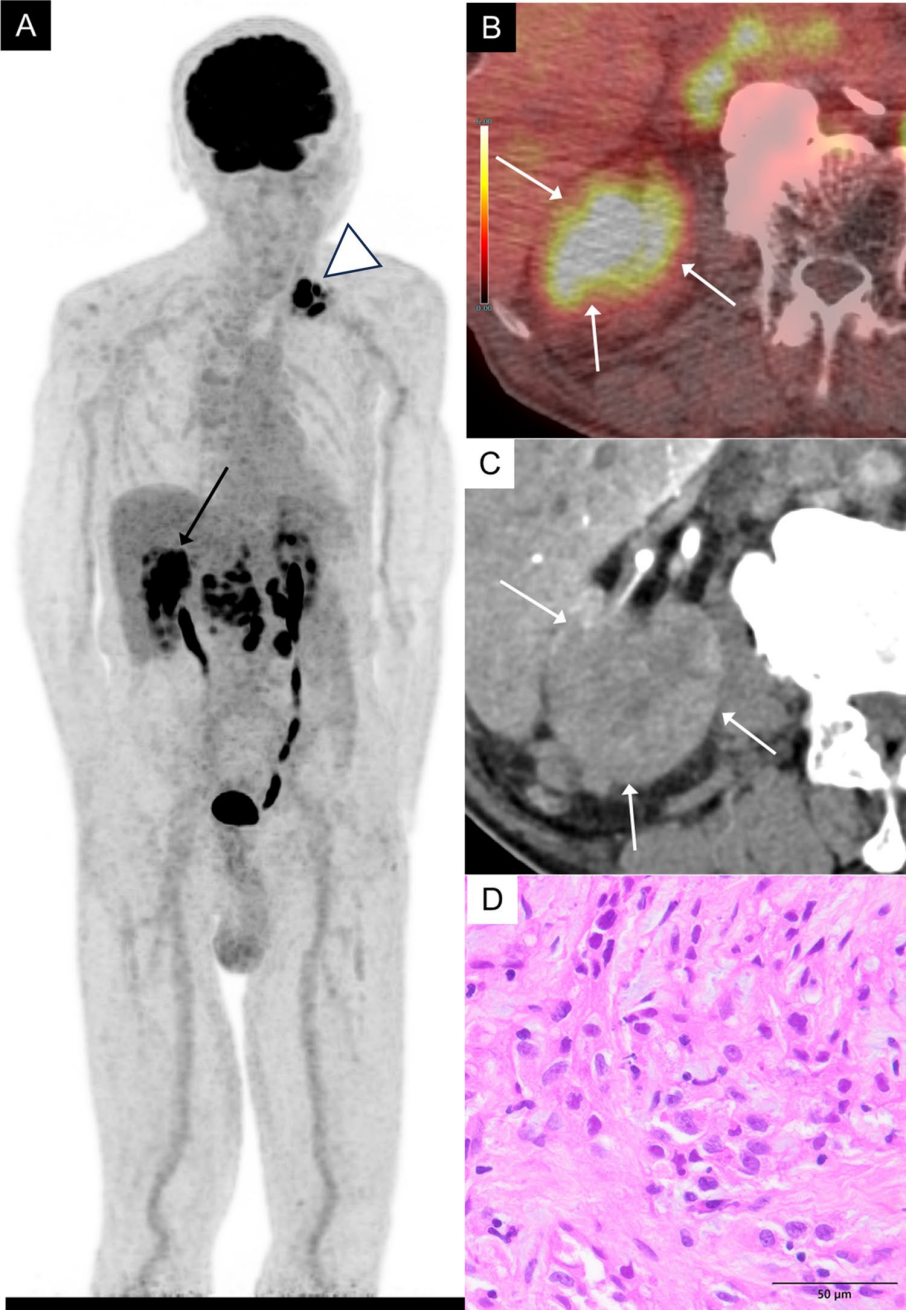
An 85-year-old man with a mucinous tubular and spindle cell carcinoma of the right kidney. A MIP of FDG-PET image shows intense mass-like FDG uptake in right kidney (arrow) and in the left subclavian lymph nodes (arrowhead). B Axial FDG-PET/CT image shows intense FDG uptake in a mass of the right kidney (arrows), showing an SUVmax 14.4, while normal renal cortex shows an SUVmax 2.7. C Axial contrast-enhanced CT in the corticomedullary phase shows a poor enhancement in the mass (arrows). D The tumor exhibits trabecular patterns with mucinous stroma. In this case, the tumor cells are more atypical than in usual cases
